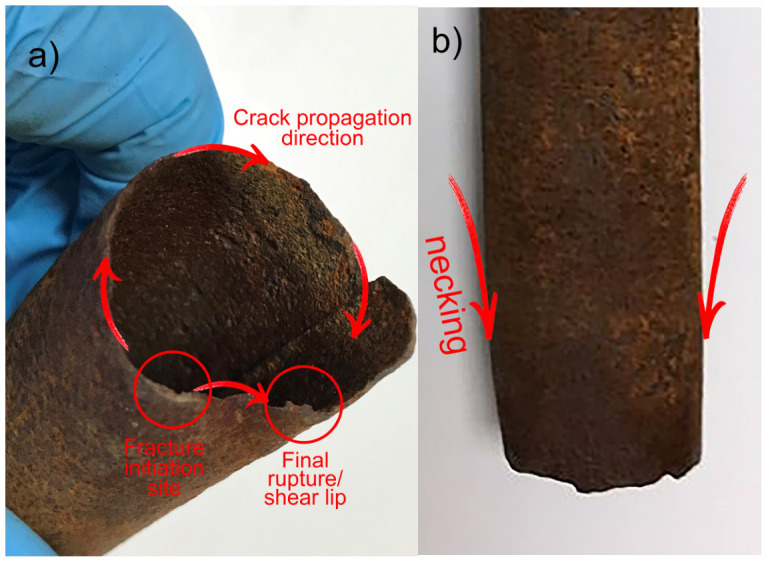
Macroscopic appearance of the failed CT-80 coiled tubing at the fracture location: (a) fracture wall showing the identified fracture-initiation site, crack-propagation directions, and final rupture region (shear lip); (b) local necking and reduction in tube diameter at the fracture site. The annotations indicate the principal macroscopic fracture features.
